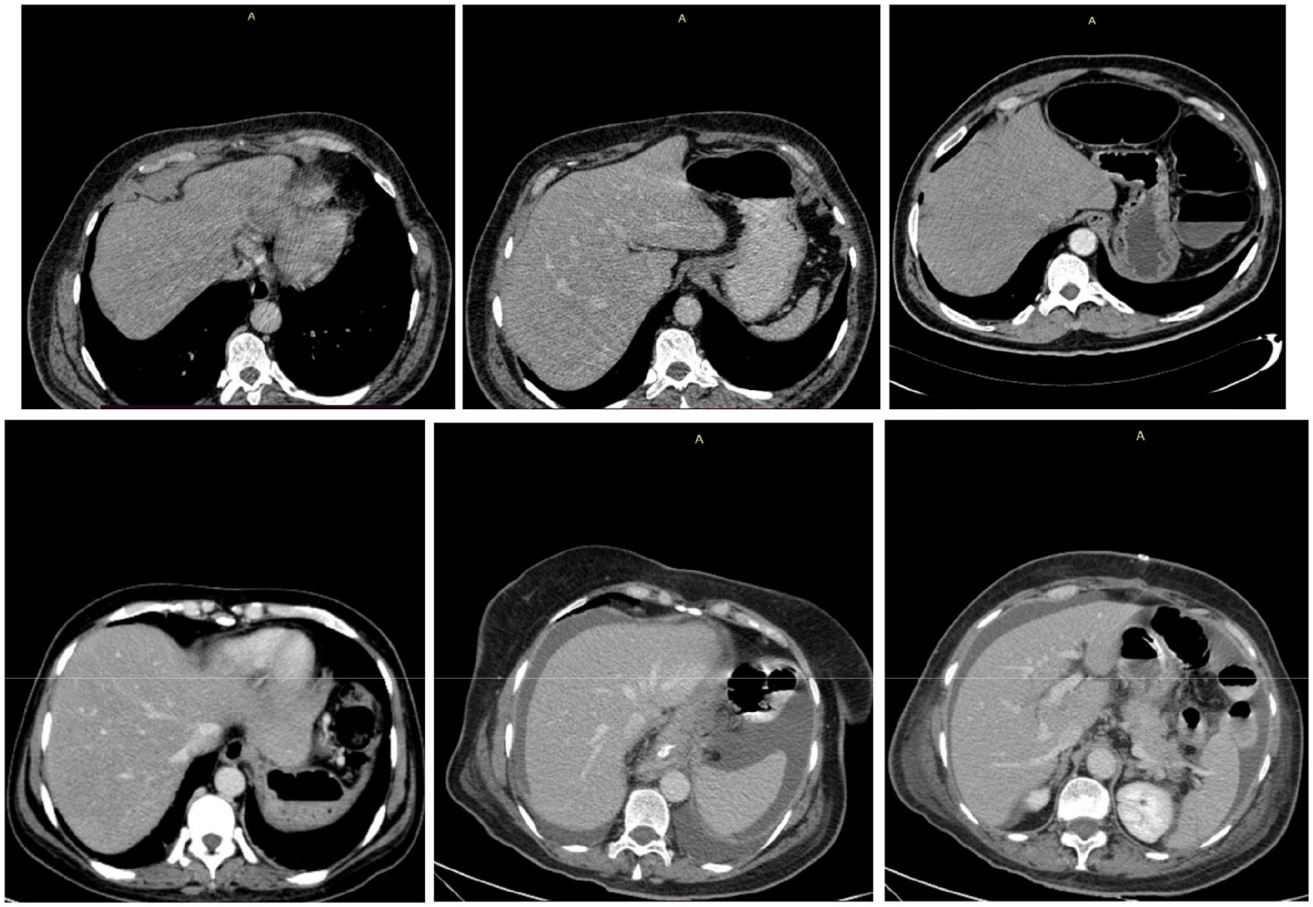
Sample of liver metastasis CT images.
